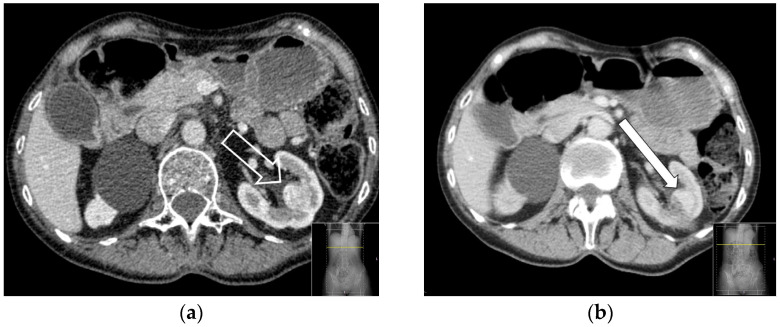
(a,b) Example of the transverse section of a contrast-enhanced CT of a patient before and after the RFA. (a) Before the RFA there is an enhanced RCC (big arrow) in the left kidney. (b) Three months later, the follow-up CT shows the remaining enhancement (long arrow) in the lateral aspect of the treated tumor (residual tumor).
